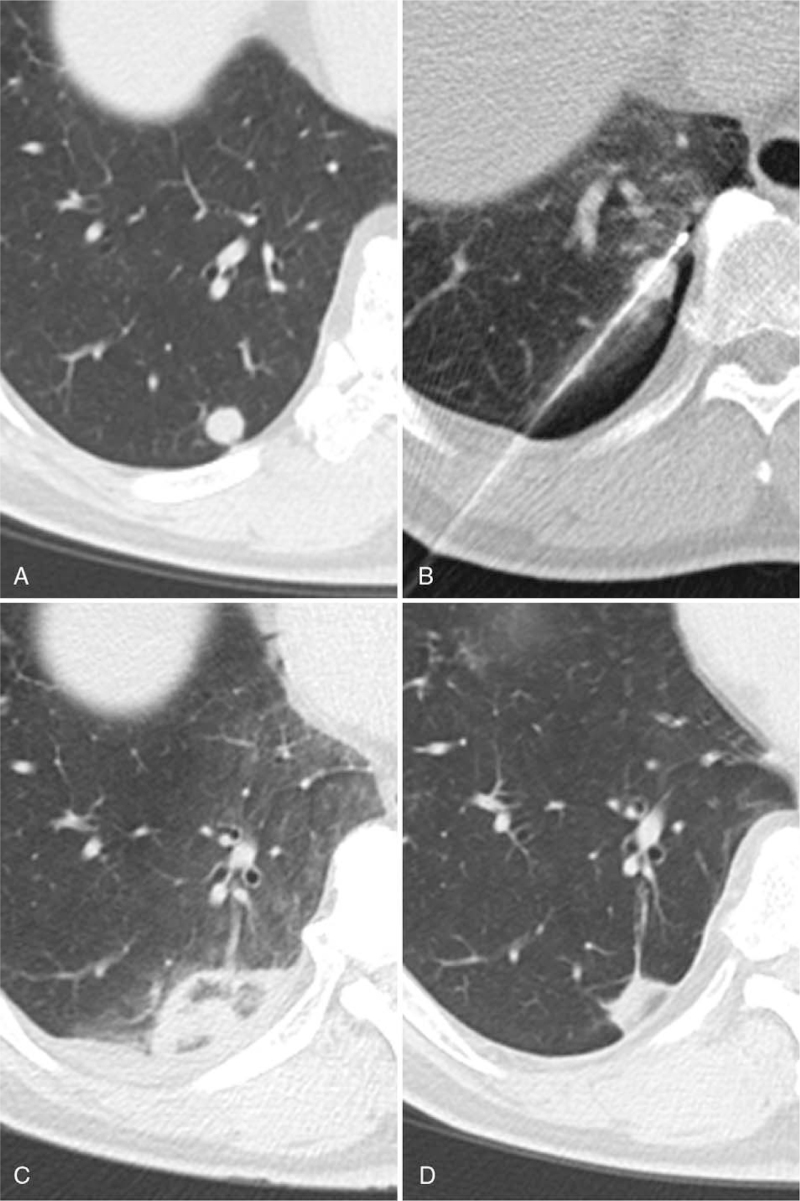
Sequential axial CT images before RFA (A), during RFA (B), at 3 days (C), 1 year (D) after RFA show dissolving of metastasis.
